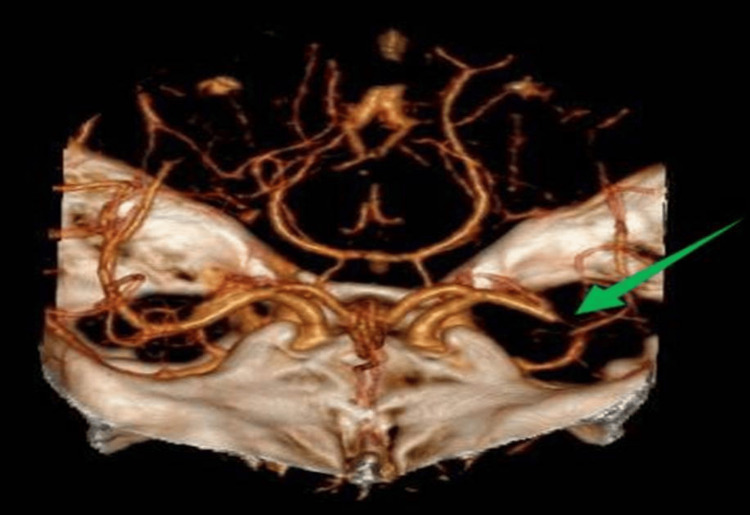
Occlusion of the M1 segment of the left middle cerebral artery, as shown by computed tomography angiography
